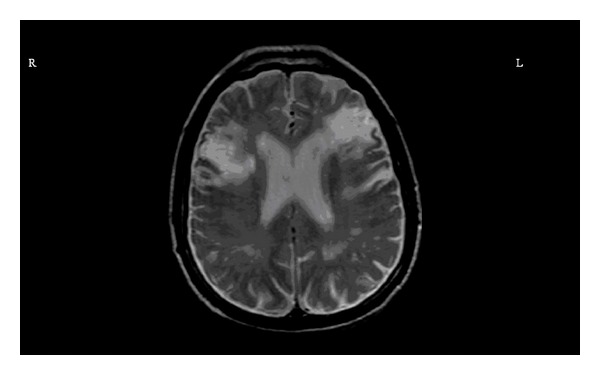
Magnetic resonance imaging sequences demonstrate bilateral inferior frontal gyrus chronic infarct (R: Right, L: Left).
